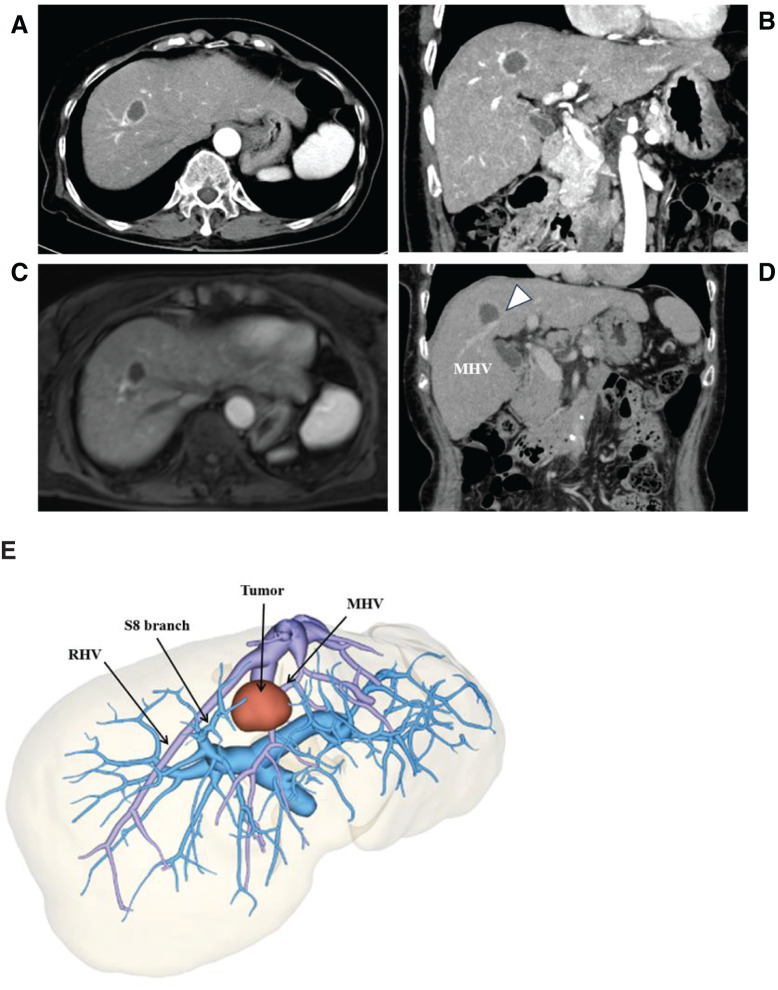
Findings of CT scan with contrast and 3D reconstruction. (A, B) Axial (A) and coronal (B) views of preoperative CT scan showing a 24-mm mass in segment 8 of the liver. (C, D) Axial (A) and coronal (B) views of the preoperative CT scan showing the tumor in segment 8 bordering the MHV (indicated by a triangle in the figure). (E) 3D reconstruction of the CT scan. MHV, middle hepatic vain; RHV, right hepatic vein
